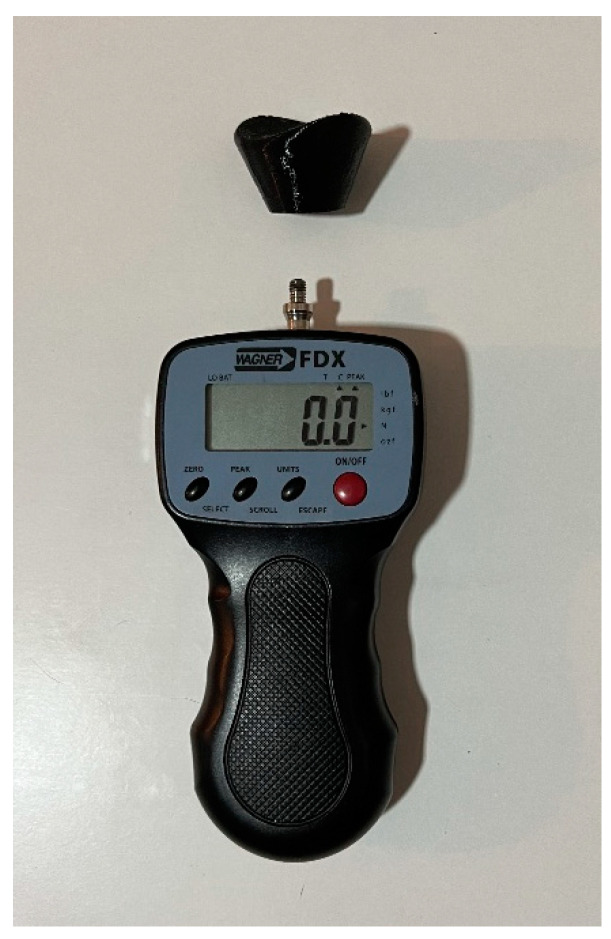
The digital force gauge with Hallux adapter (FPX^®^ 25, Wagner Instruments^®^, Greenwich, CT, USA).
